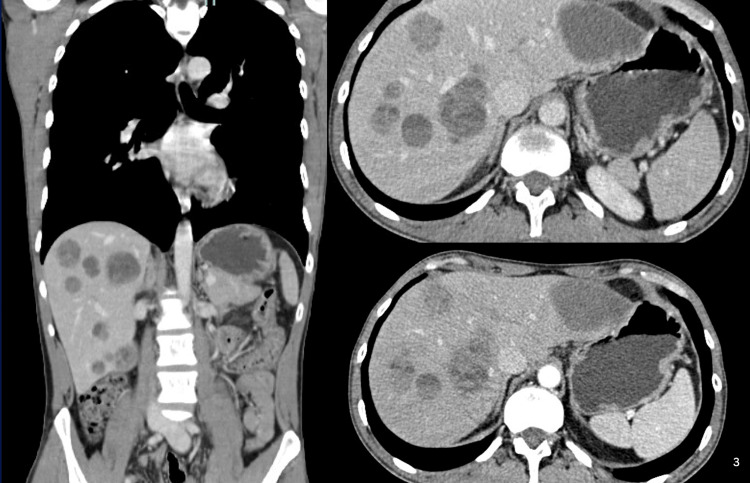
Contrast-enhanced CT imaging showing bilobar hepatic metastases Abdominal CT images (coronal view on the left and axial views on the right) showing a dominant lesion in the left hepatic lobe (segments II–III), measuring approximately 6 cm. Additional smaller lesions are visible, consistent with bilobar hepatic metastases.
